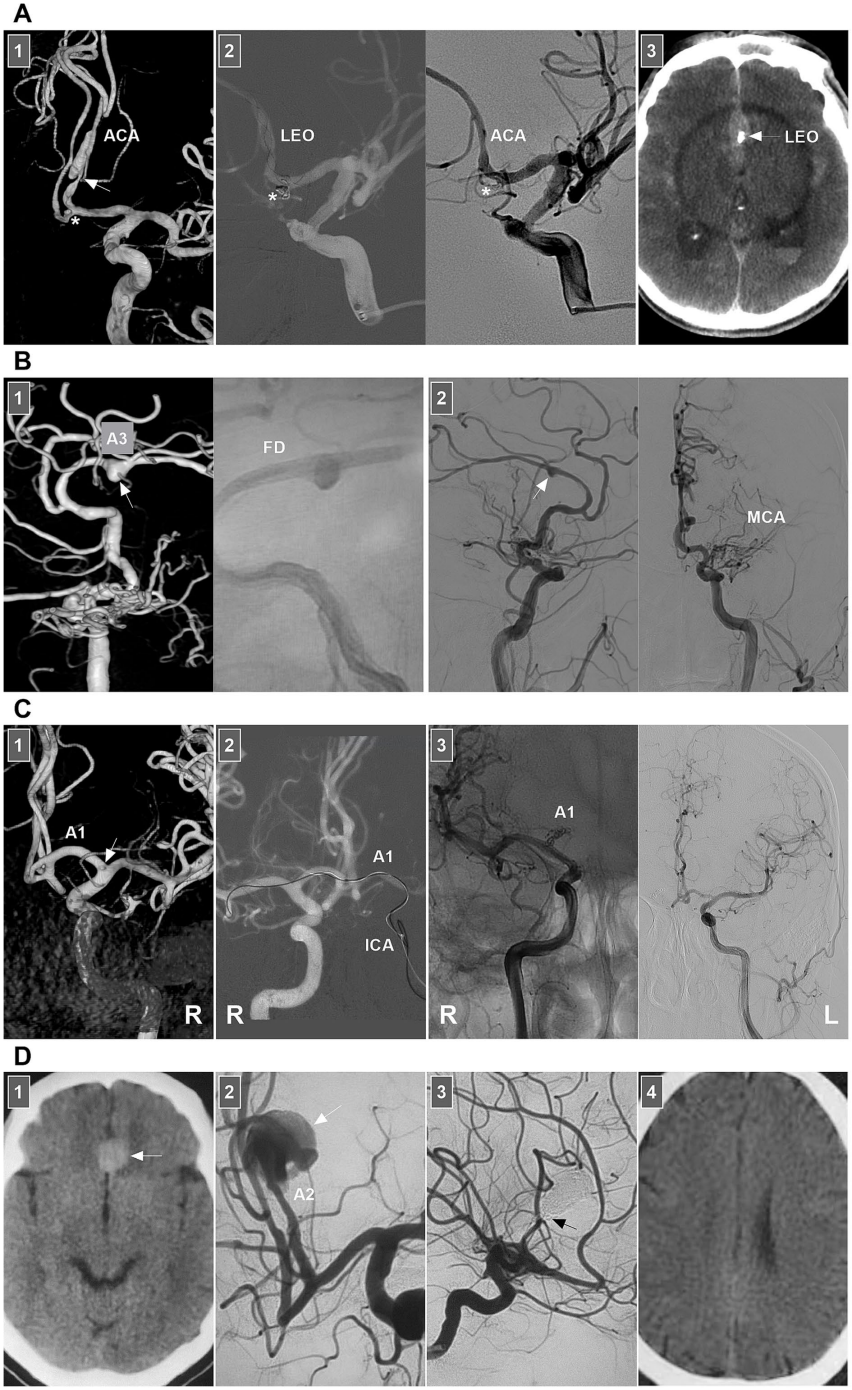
EVT of ACA dissections. (A) Panel 1: DSA showing a typical ruptured dissection (arrow) at A2 segment trunk and an AcomA aneurysm (asterisk). Panel 2: Roadmap image (left panel) and DSA (right panel) showing that the AcomA aneurysm was coiled (asterisks) and the dissection was reconstructed by LEO baby stenting. Panel 3: Postoperative X-per CT showing the LEO stent (arrow) was in the hematoma of anterior longitudinal fissure, indicating the ACA dissection was ruptured. (B) Panel 1: DSA (left panel) showing a fusiform A3 dissection (arrow), X-ray image (right panel) showing that the A3 dissection was covered by FD. Panel 2: Four-month follow-up DSA (left panel) showing the A3 dissection regressed, DSA showing the previous MCA occlusion. (C) Panel 1: DSA showing a small dissection at right A1 origin (arrow). Panel 2: Roadmap image showing that the contralateral trans-circulation approach to treat the dissection by occluding A1 origin. Panel 3: Six-month follow-up DSA (left and right panels) showing that right A1 origin was occluded, left ACA supplied the bilateral ACAs. (D) Panel 1: CT showing a lesion (arrow) in the anterior longitudinal fissure. Panel 2: DSA showing a giant A2 dissecting aneurysm (arrow). Panel 3: DSA showing the aneurysms was trapped and the proximal A2 was occluded (arrow). Panel 4: Postoperative CT showing no ischemic finding in the frontal lobe. ACA, anterior cerebral artery; A1, first segment of the ACA; A2, second segment of the ACA; A3, third segment of the ACA; AcomA, anterior communicating artery; CT, computed tomography; DSA, digital subtraction angiography; EVT, endovascular treatment; FD, flow diverter; ICA, internal carotid artery; L, left; R, right.
